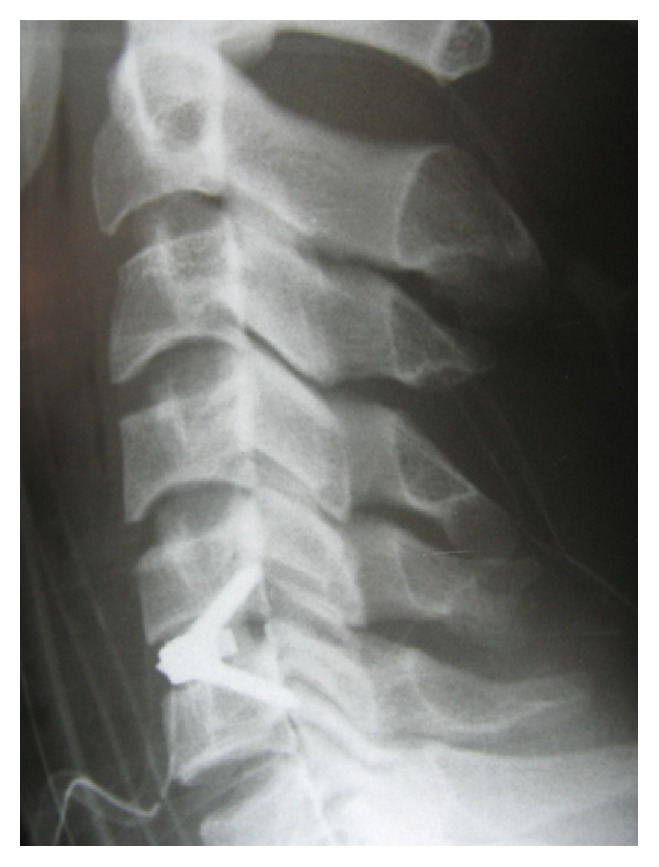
Postoperative X-ray of ZeroP.
